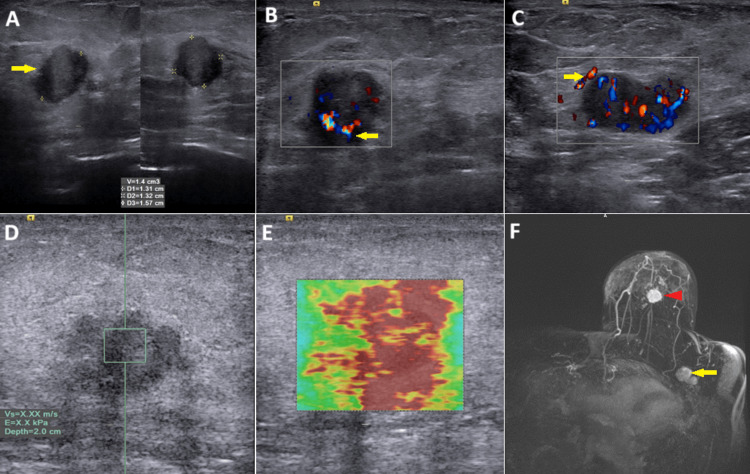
Pre-ablation images. (A) Greyscale ultrasound showing an irregularly edged hypoechoic lesion at about 6 o'clock position of the left breast (arrow). (B) Doppler ultrasound of the primary lesion showing moderate vascularity (arrow). (C) Greyscale ultrasound with color Doppler interrogation showing metastatic appearing left axillary lymph node with marked vascularity (arrow). (D) Quantitative ultrasound elastography showing very high stiffness above the default upper limit of SWE velocity (≥6.5 m/s) indicated as X.XX. (E) Qualitative ultrasound elastography showing very high stiffness evidenced by a predominately red color map. (F) Post-contrast dynamic MRI with subtraction MIP showing the primary lesion (arrowhead) and ipsilateral metastatic lobulated axillary lymph node (arrow) SWE: shear wave elastography; MIP: maximum intensity projection
